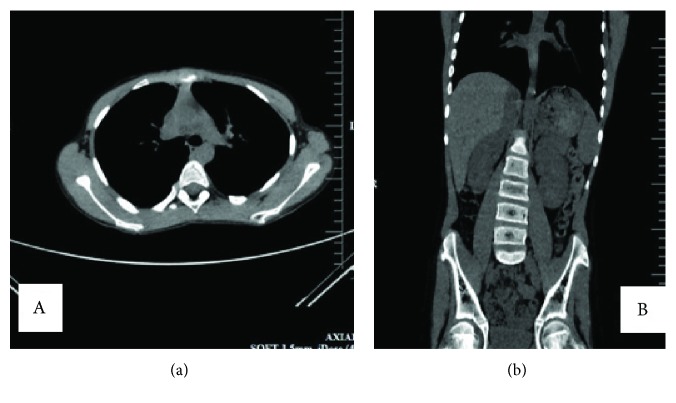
CT scan showing (a) axial section of the chest and pelvis cavity, no metastases detected, and (b) abdomen and chest are normal.
